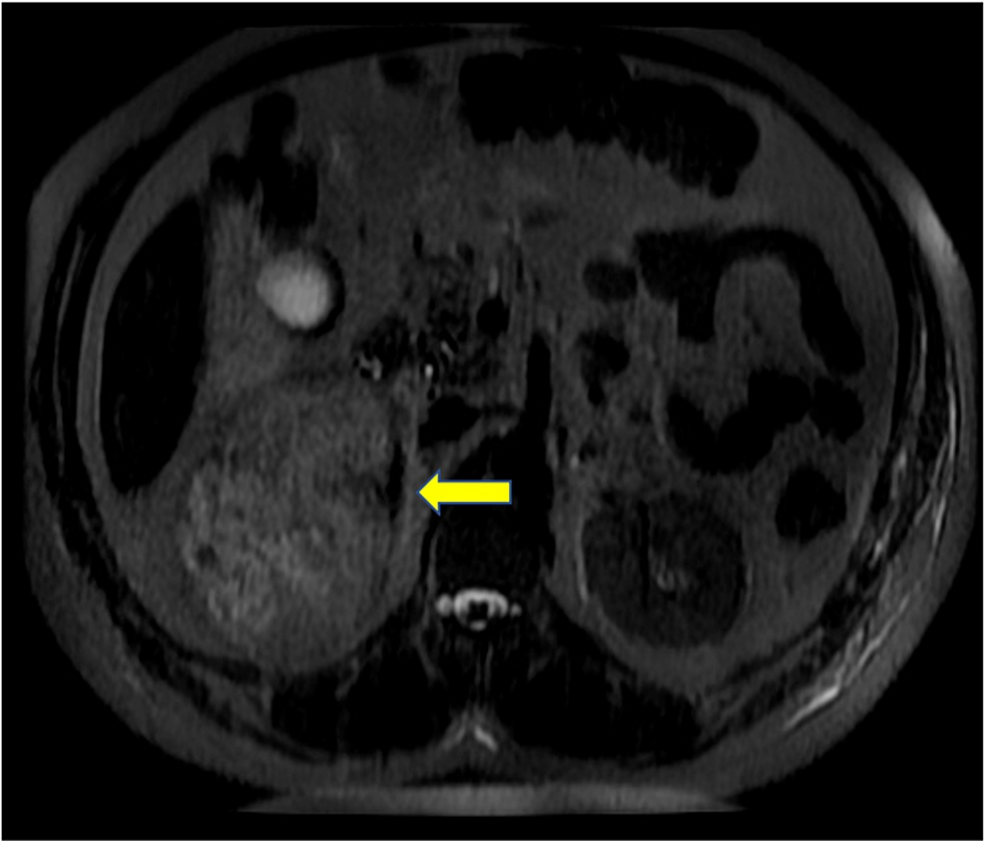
MRI T2 Single Shot Fast Spin Echo - Axial View Image shows areas of focal hemorrhage with blooming (yellow arrow).
